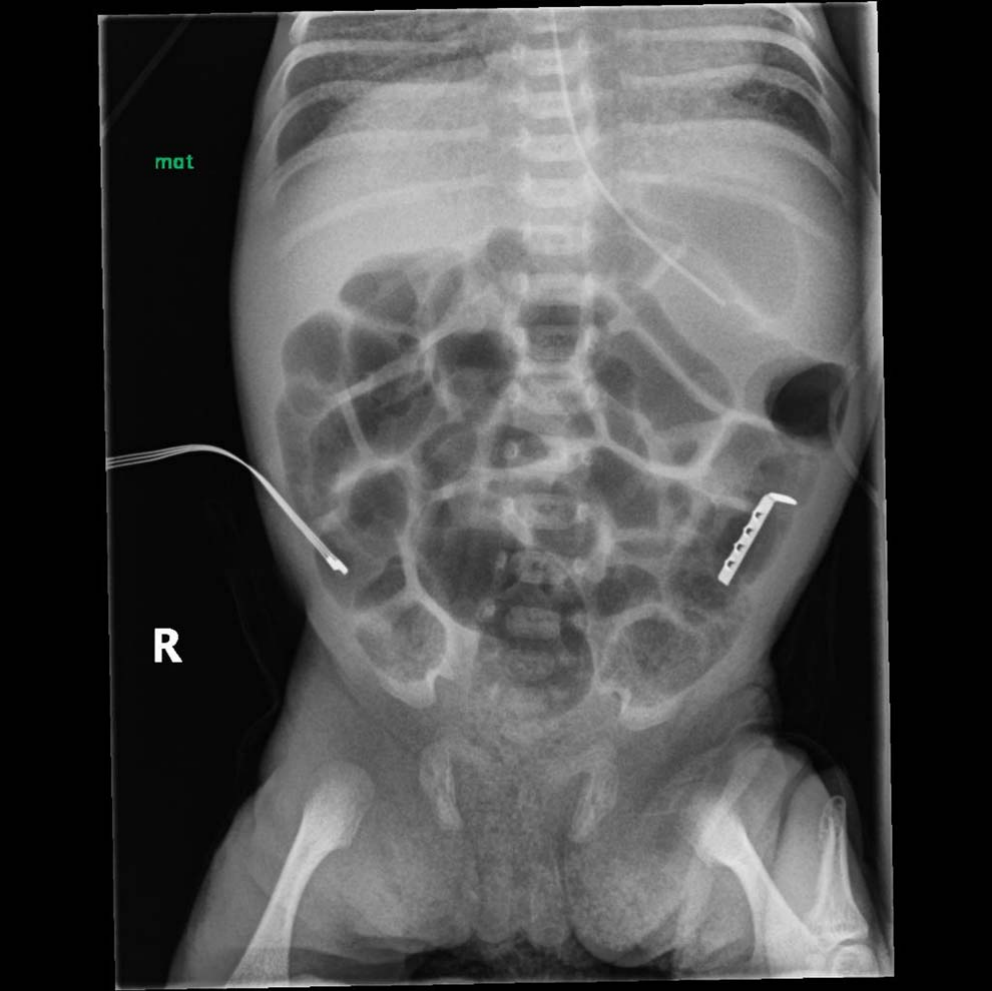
Abdominal x-ray shows the presence of an L-shaped object overlying the left abdomen 11 days after admission.
